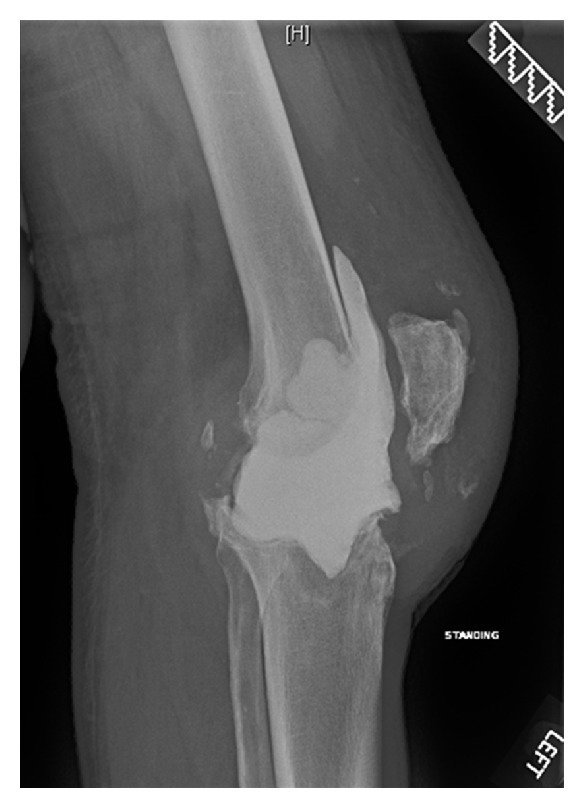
Lateral X-ray of the left knee after the first-stage revision with an antibiotic-impregnated cement spacer 6 months after initial TKA.
